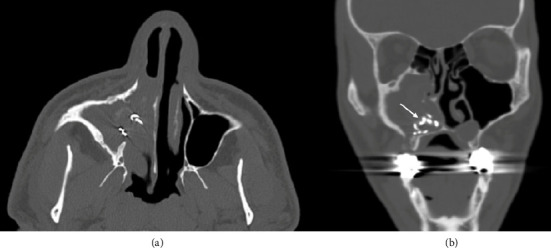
Axial (a) and coronal (b) noncontrast CT of the sinuses showing complete opacification of the right maxillary sinus and anteroinferior nasal cavity, irregular-shaped radio-opaque materials in the floor of the antrum consistent with retained foreign body (arrow), evidence of prior inferior meatal antrostomy to the maxillary sinus, and oroantral fistula.
